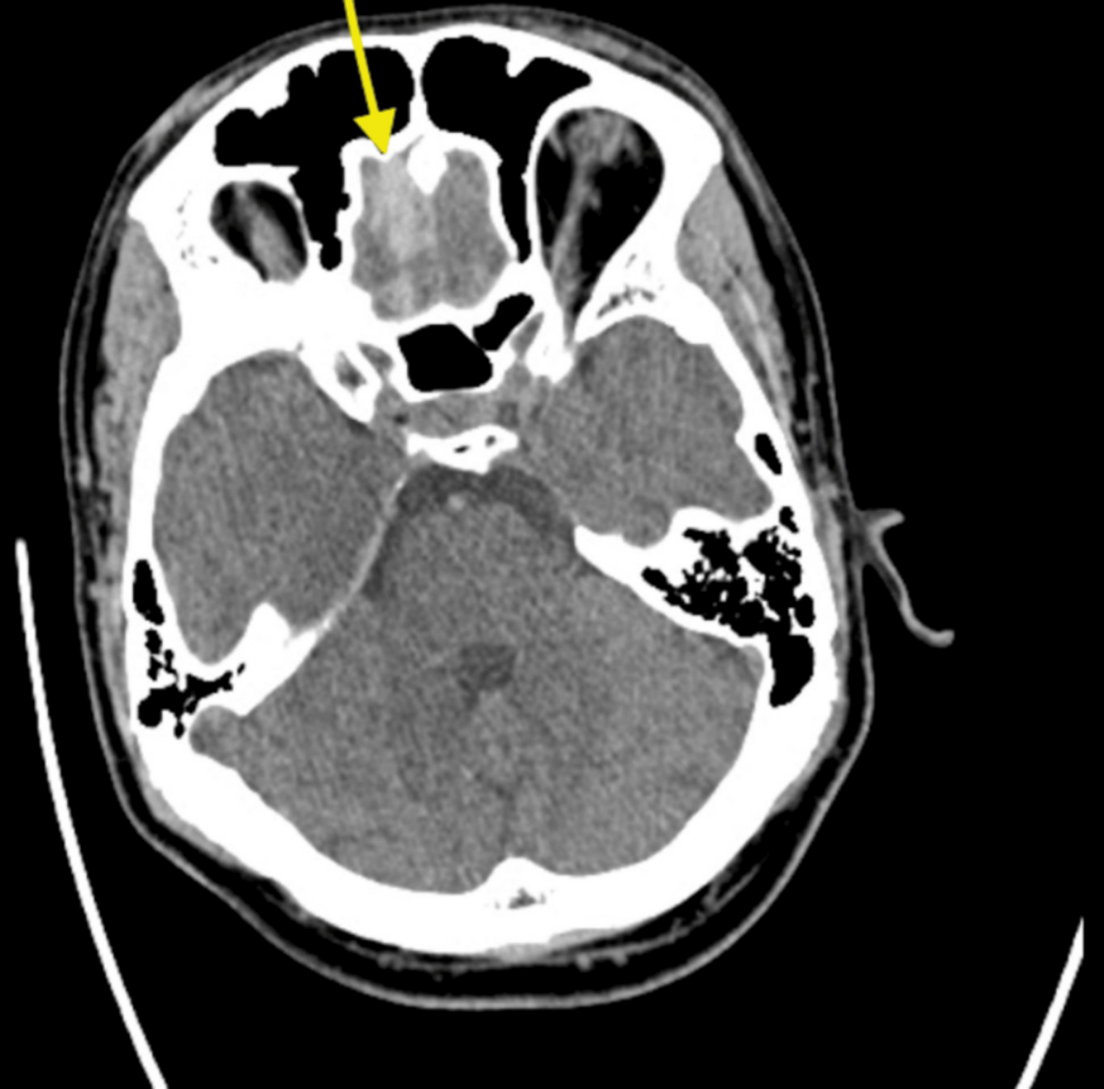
Brain contusion Axial noncontrast CT brain showing a small right frontal brain contusion along the gyrus rectus (yellow arrow). These findings may be compatible with a contrecoup pattern in the appropriate clinical context
